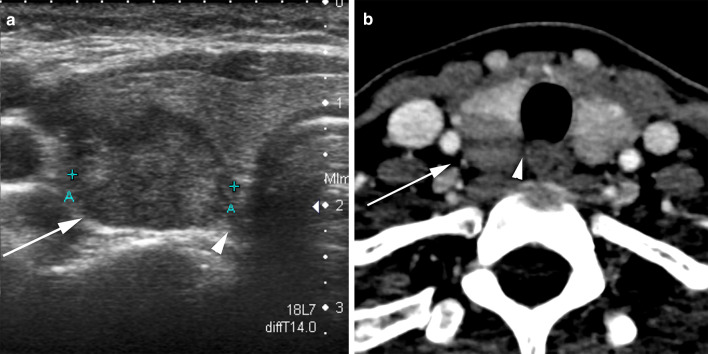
40-Year-old female with PTC in the posterior aspect of right lobe of thyroid gland. Transverse ultrasound image (a) shows a 13 mm PTC with loss of intervening normal thyroid parenchyma between the PTC and anatomic course of recurrent laryngeal nerve (RLN) (arrowhead). Corresponding axial contrast enhanced CT scan at the level of the PTC (b) again confirms the absence of intervening normal thyroid parenchyma between the PTC and the expected course of RLN (arrowhead) with protrusion of the nodule posteriorly beyond the margin of the gland. The patient was therefore not offered AS in our study
